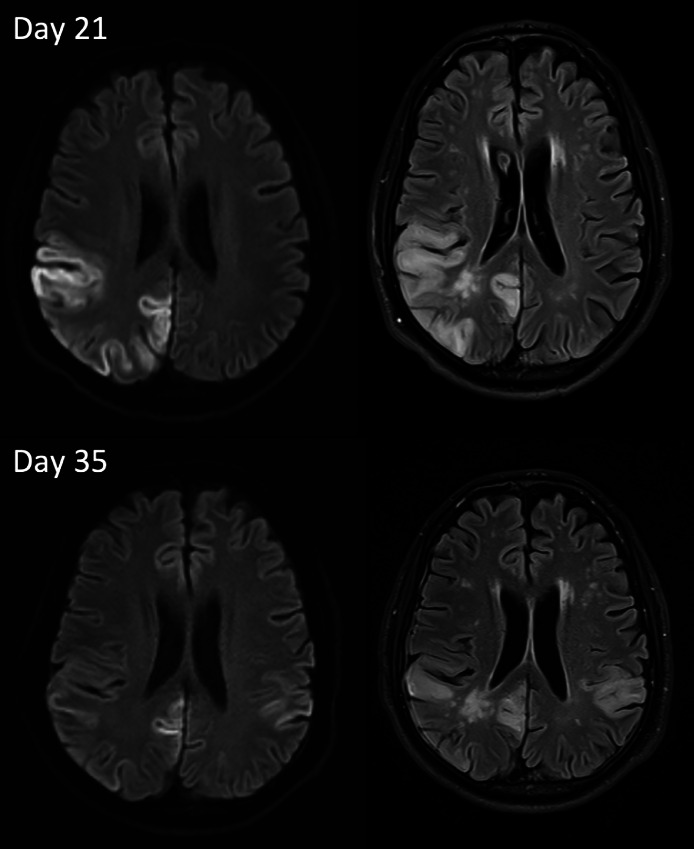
Diffusion restriction and FLAIR images from Day 21 and Day 35 show right MCA and PCA territory cortical based diffusion restriction, not corresponding to a single vessel disease and suggestive of possible nonvascular source.
